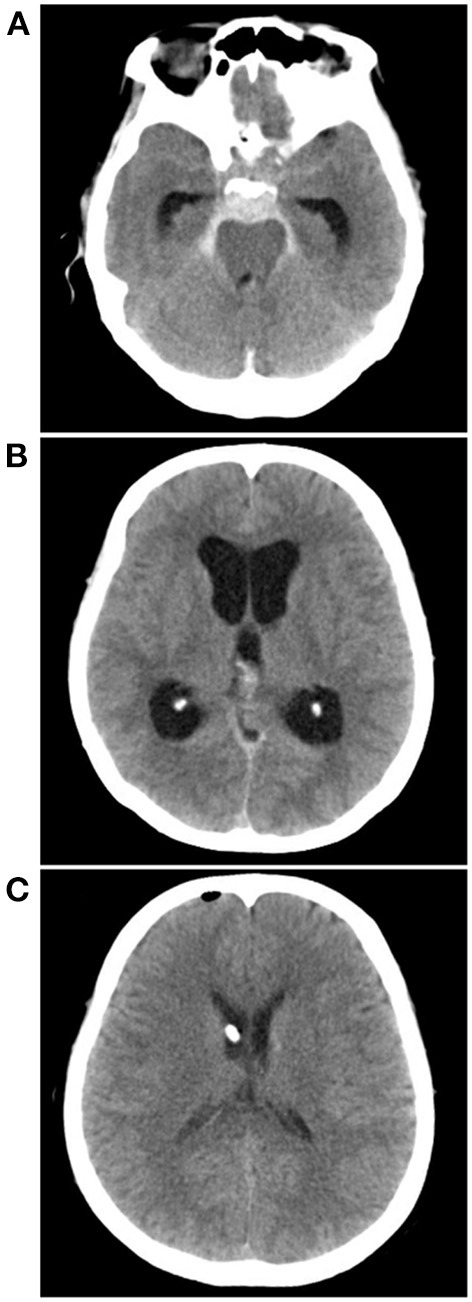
External ventricle drainage in a PNSAH. (A) CT showing a classic PNSAH. (B) CT showing acute lateral ventricle dilation. (C) CT showing the normal size of lateral ventricle after external ventricle drainage. CT, computed tomography; PNSAH, perimesencephalic non-aneurysmal subarachnoid hemorrhage.
